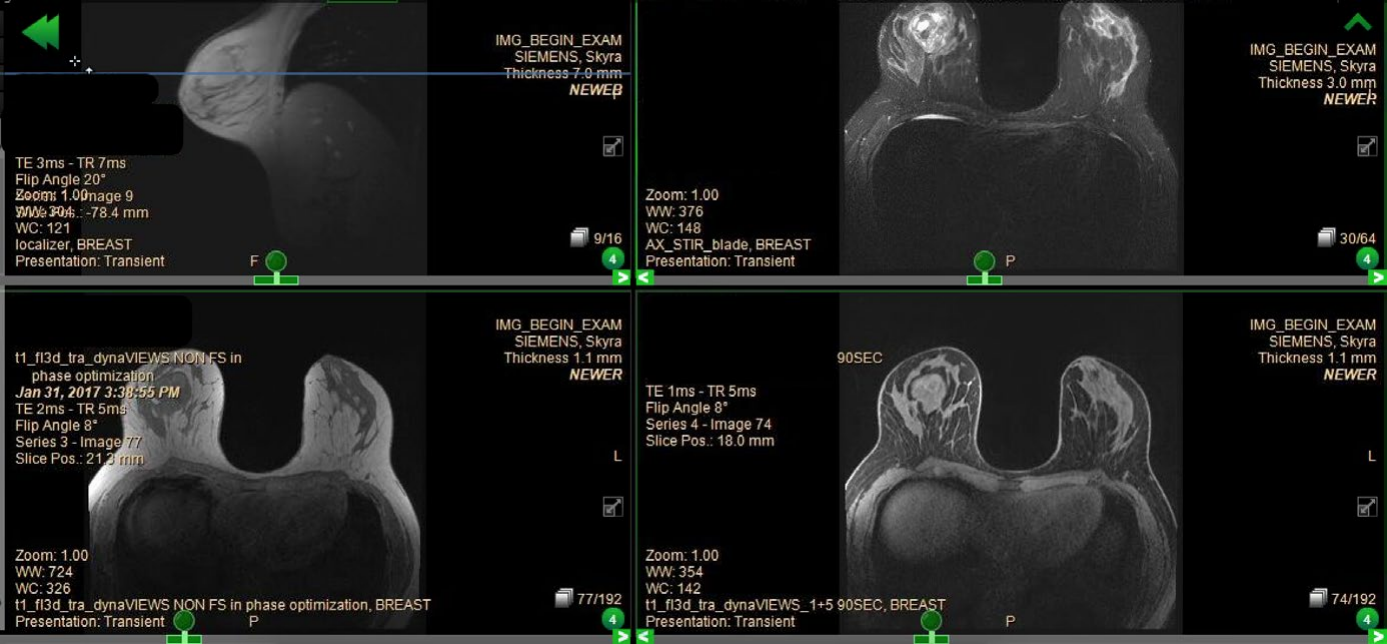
MRI of breast showing a large right breast mass
